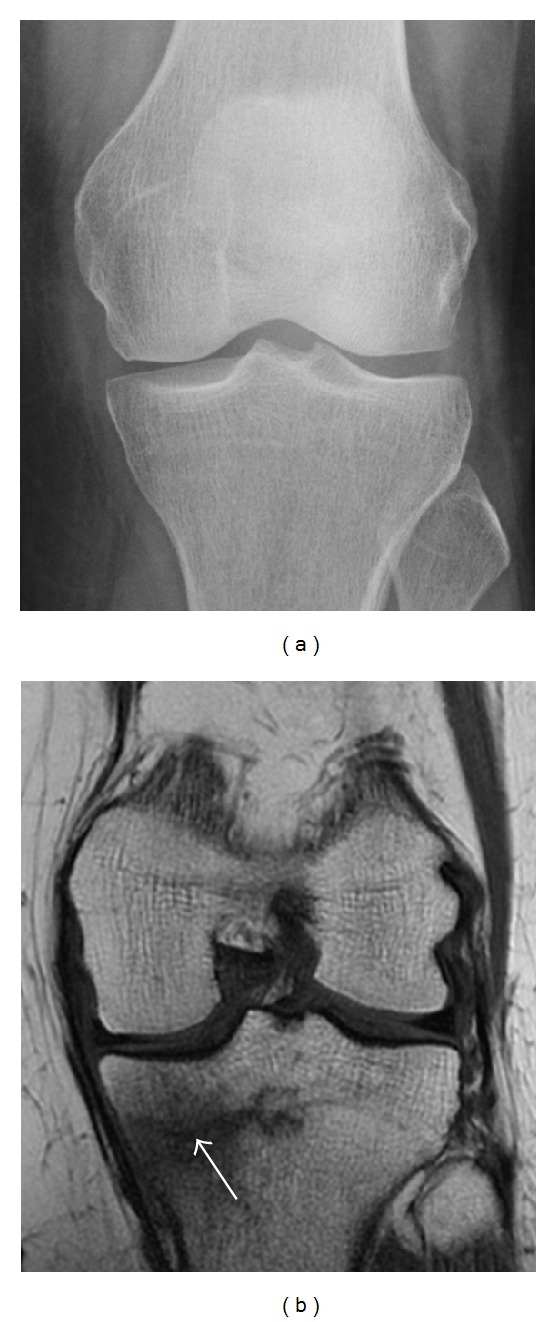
Proximal metaphyseal fatigue fracture of the tibia in a 27-year-old recent male military recruit. (a) Anteroposterior radiograph is within normal limits. (b) Coronal T1-weighted MR image shows a marked linear hypoattenuation along the medial tibial metaphysis (arrow) surrounded by diffuse hypointensity in keeping with posttraumatic edema.
